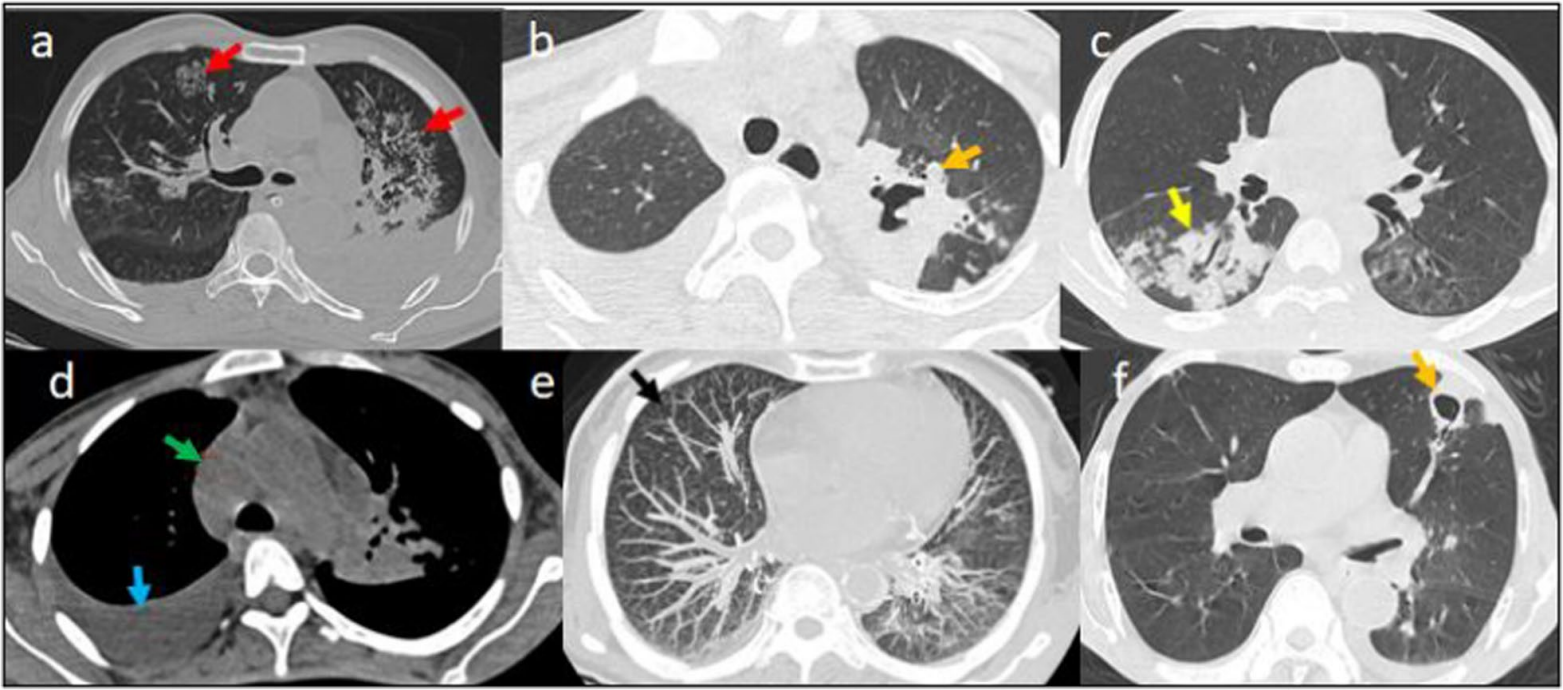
HRCT chest axial sections of different patients showing imaging features of COVID patients mimicking tuberculosis—a showing multiple tree in bud nodules (red arrow) in bilateral upper lobes (left > right). b Cavity with surrounding consolidation (orange arrow) in apicoposterior segment of left upper lobe. c Patchy dense consolidation (yellow arrow) in superior segment of right lower lobe. d Enlarged right paratracheal node with right pleural effusion. e MIP image showing multiple tiny centrilobular nodules (black arrow). f Small cavity with surrounding consolidation (orange arrow) in lingular segment of left upper lobe and showing communication with segmental bronchus
